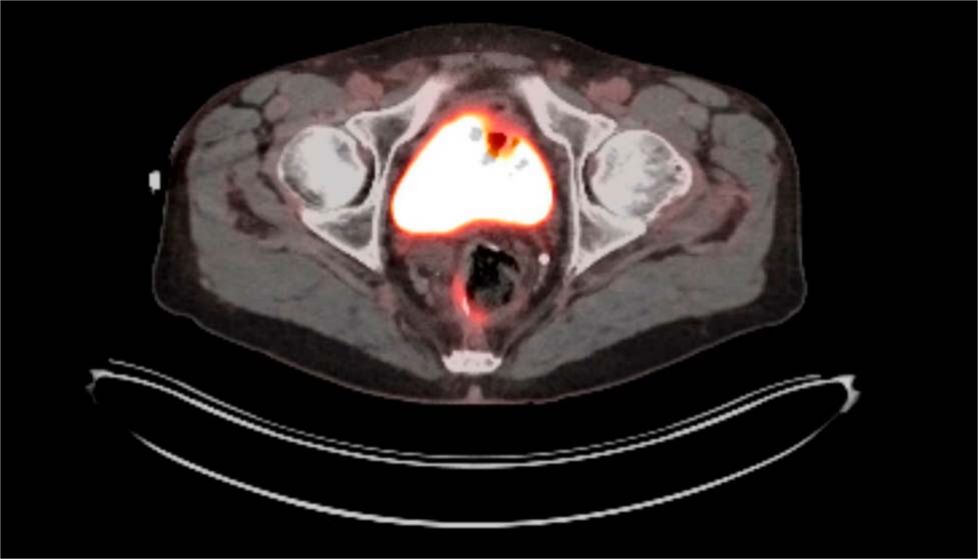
Repeat PSMA positron emission computed tomography imaging showing postsurgical changes from the radical prostatectomy and J-pouch creation with moderate PSMA expression along the right posterolateral margin of the J-pouch that was compatible with metastasis. PSMA, prostate-specific membrane antigen.
